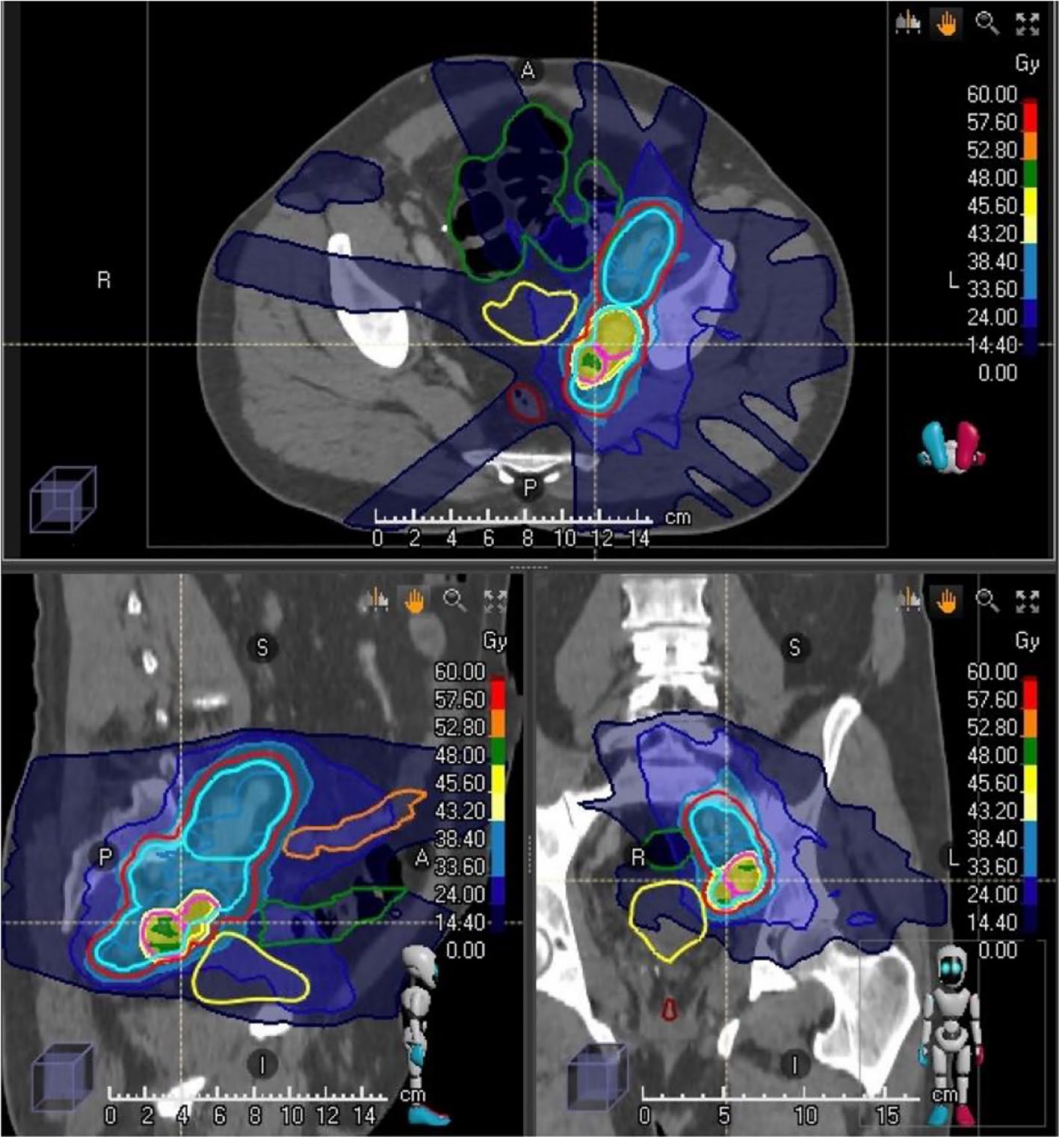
Radiation dose distribution of high-dose treatment of the PSMA-PET-positive nodal volume at ablative dose in 12 fractions as simultaneous integrated boost within elective dose to the entire lymphatic region. Dose in Gray as color-coded on the right-hand scale
